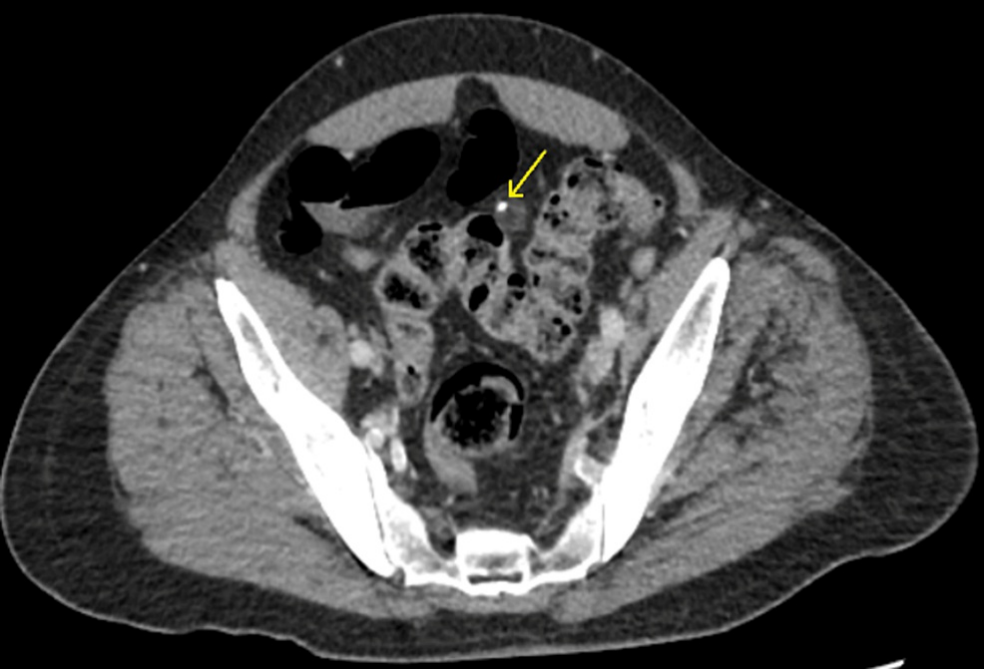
CT showing EA. The yellow arrow points to the involved epiploic appendage of the sigmoid colon. EA: Epiploic appendagitis
